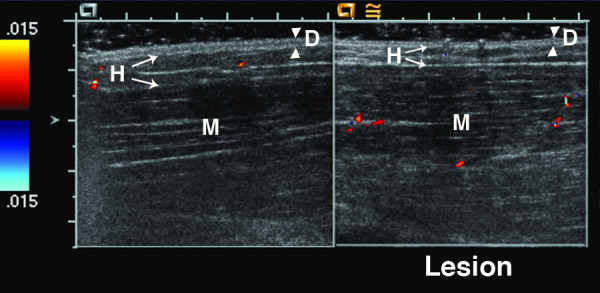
15 year old girl with over an 8-year history of localized scleroderma. She initially presented with linear scleroderma of one hand that extended up her arm. There has been recent extension of this lesion to her upper back, and circumscribed morphea lesions have developed on her chest and thigh. Ultrasound image shown is of her upper arm, which had chronic atrophy and hyperpigmentation, but also mild erythema. There is increased vascularity in the lesion muscle, with over 10 discrete color Doppler signals in this layer versus none in the normal muscle. The deep tissue vascularity is scored as 2 because there are over two-fold more color Doppler signals in the lesion compared to normal; the vascularity is not scored as 3 because all of the lesion color Doppler signals are of a similar small size. The normal hypodermis has over two-fold more color Doppler signals than the lesion in this image, giving a lesion hypodermal vascularity score of -1. D = dermis, H = hypodermis, and M = muscle. Arrowheads indicate boundaries of dermis, while arrows indicate boundaries of hypodermis.
